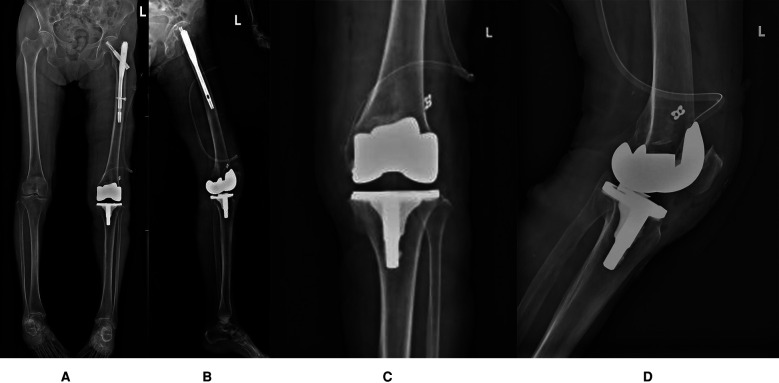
(A) Full-length frontal radiograph of the lower limb (Postoperative radiograph); (B) Full-length lateral radiograph of the lower limb (Postoperative radiograph); (C) Frontal radiograph of left knee joint (Postoperative radiograph); (D) Lateral radiograph of left knee joint (Postoperative radiograph).
